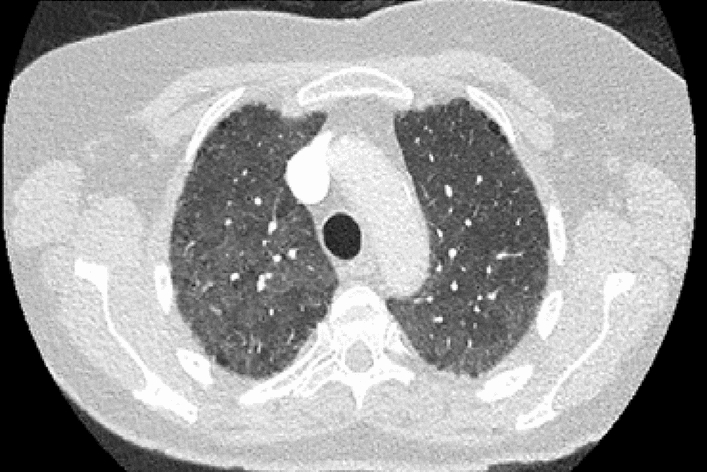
Transverse computed tomography (CT) image of chest showing diffuse ground glass inflammatory change in a case of MTXip in a patient with rheumatoid arthritis. Black areas are unaffected lung. There was no zonal predilection
